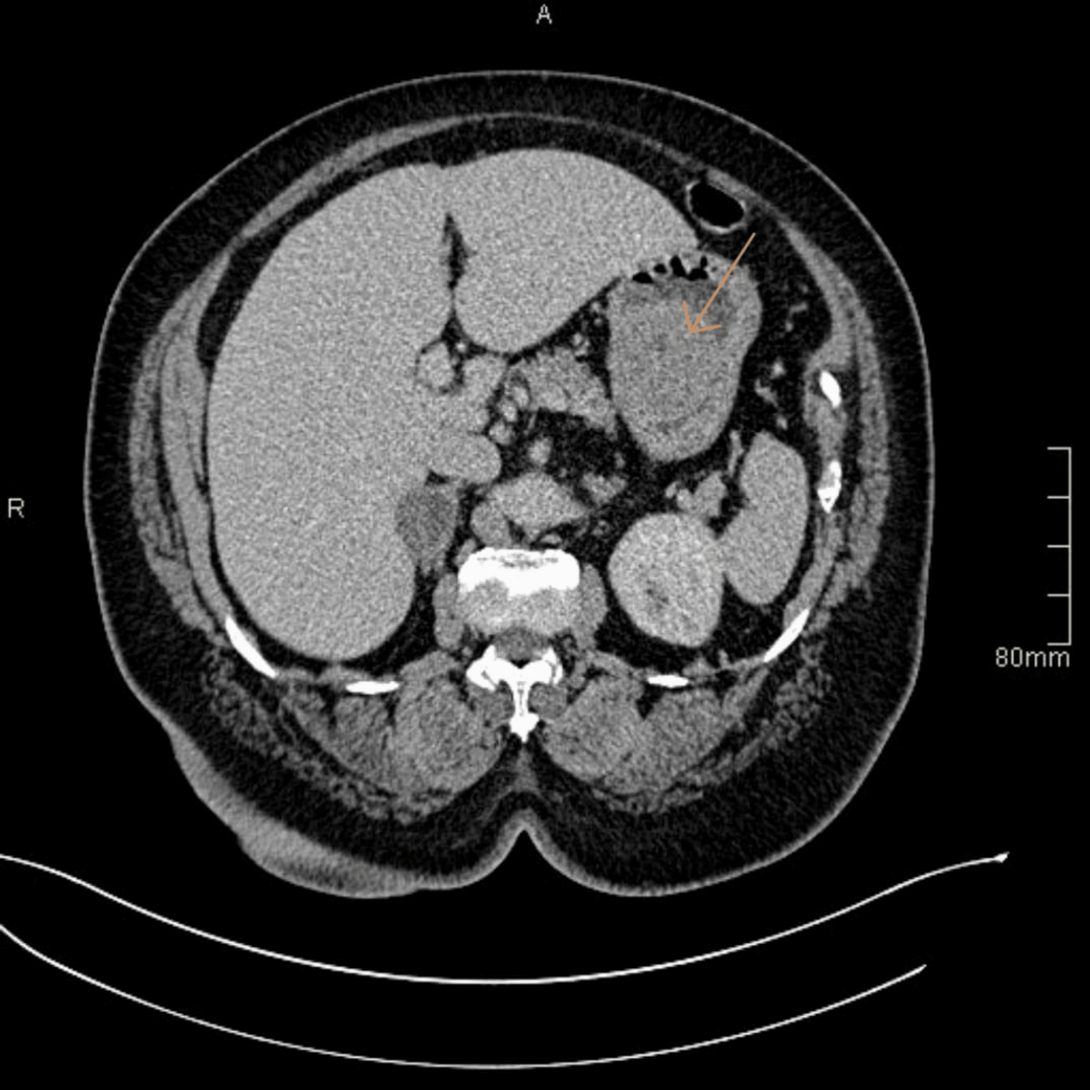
A CT of the abdomen shows a gastric mass on the lesser curvature, rounded without invasion or lymphadenopathy, measuring about 5 x 6 cm CT: computed tomography
